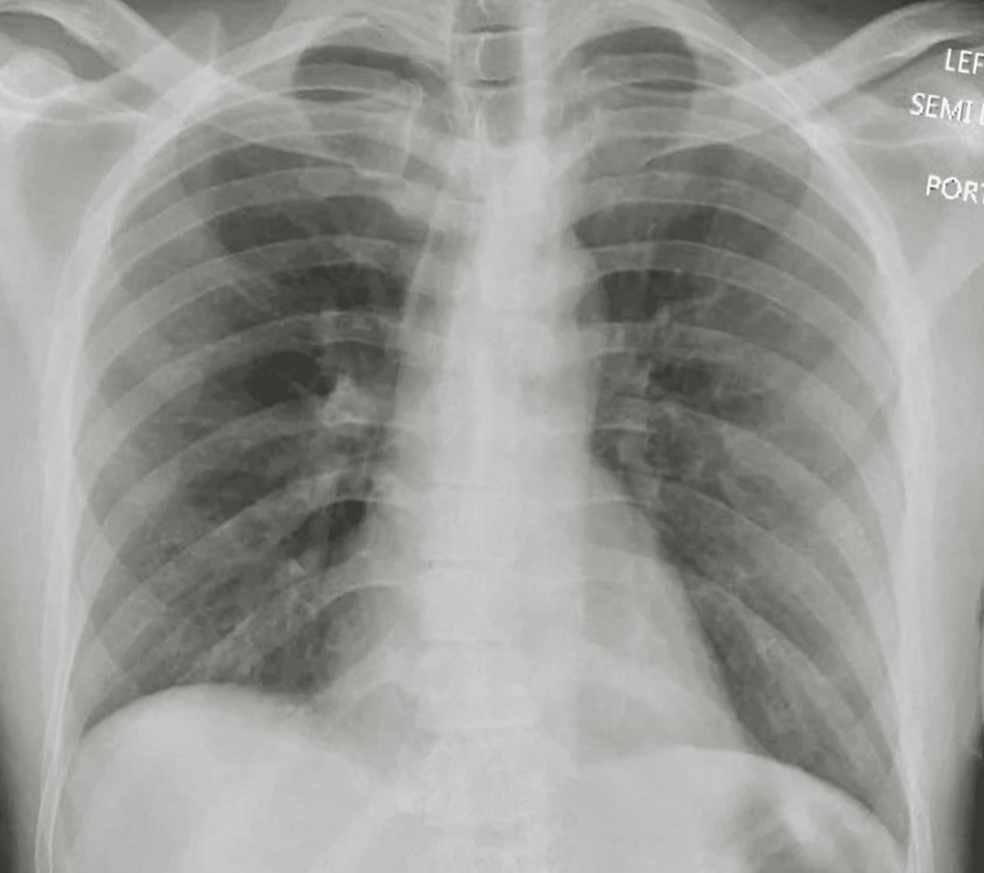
X-ray chest on day six of admission showing resolution of bilateral opacities.
